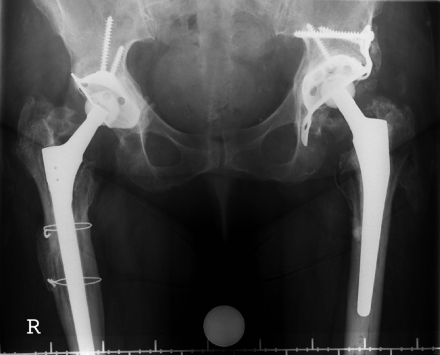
A 59-year-old woman with bilateral PI following *Staphylococcus aureus* sepsis, 2 years after bilateral 1-stage exchange. On the right side: reconstruction with a Müller reinforcement ring for a type-1 defect, and a long Wagner stem by transfemoral approach. On the left side: a reconstruction with a Burch-Schneider ring to bridge a type-2B defect. A cemented Virtec stem with transgluteal approach was implanted. At the 2-year follow-up, the HHS (bilateral) was 97, there was a slight limp, there were no radiographic signs of loosening, the transfemoral osteotomy was healed, and the infection was cured.
